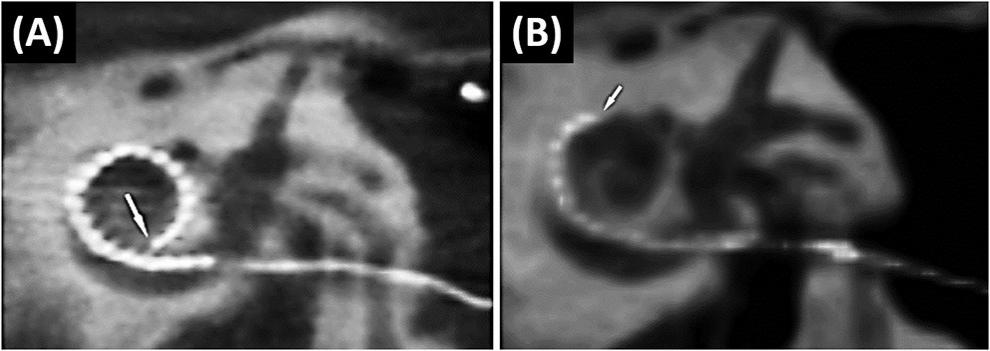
The immediate post-operative CT scan shows a fully inserted Cochlear Slim Straight array (CI422) with an insertion angle of 390°(A). The follow-up scan shows a substantially retracted electrode with six extra-cochlear electrodes and an insertion angle of 210°. The arrow points to the tip of the electrode (21) (B). Reproduced by permission of Springer Nature.
